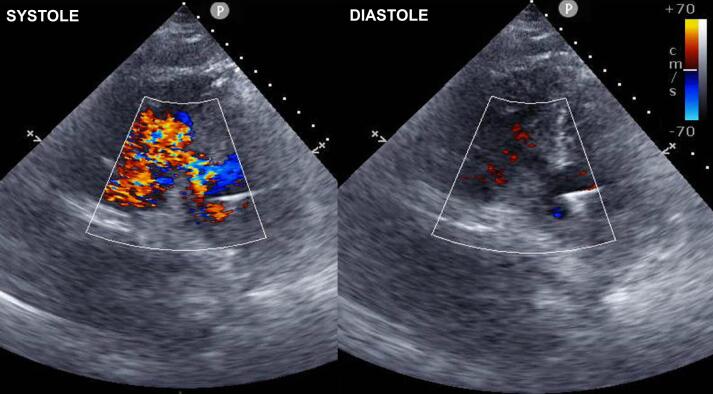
Transthoracic echocardiography before ECMO implantation. Transthoracic echocardiography shows left-to-right shunt in systole and no right-to-left shunt in diastole.
